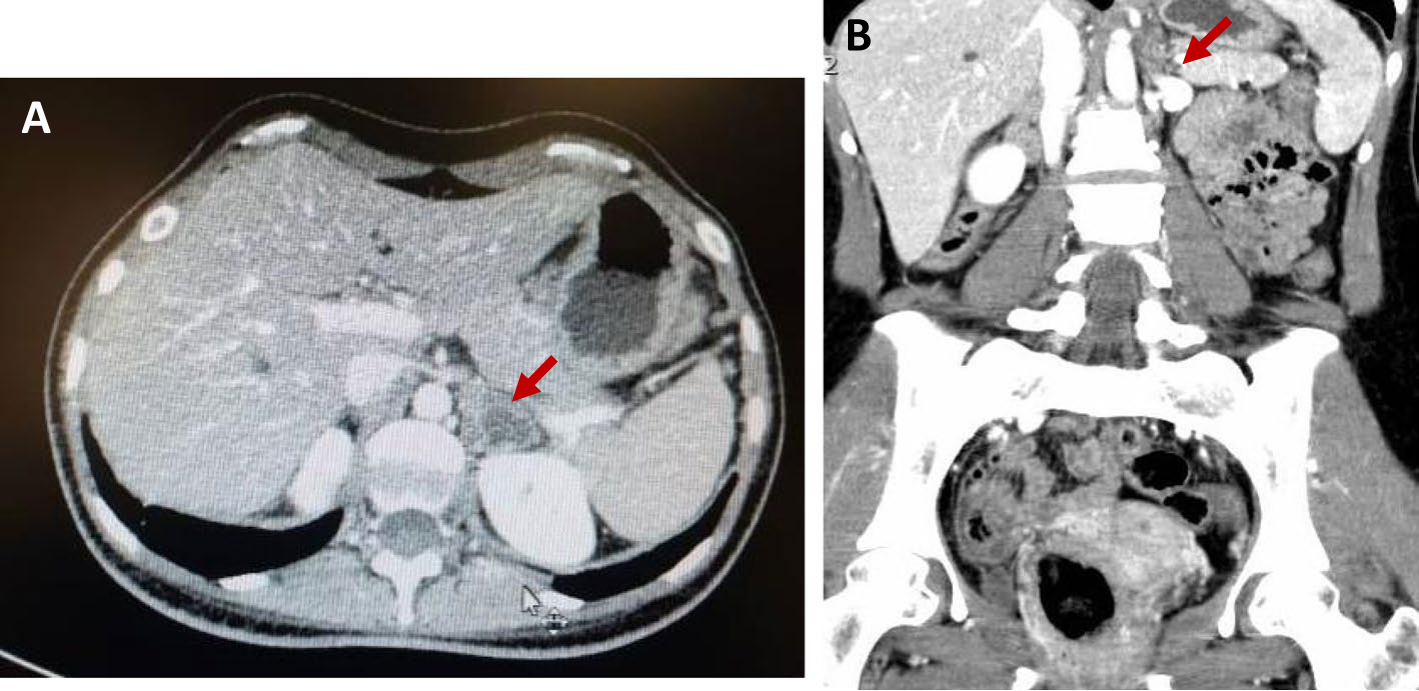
A computed tomography scan of the abdomen and pelvis of the first case with contrast shows left adrenal thick wall cystic mass with an enhanced peripheral rim. (A) The transverse view and (B) the coronal view. The red arrows indicate the areas of fat stranding
